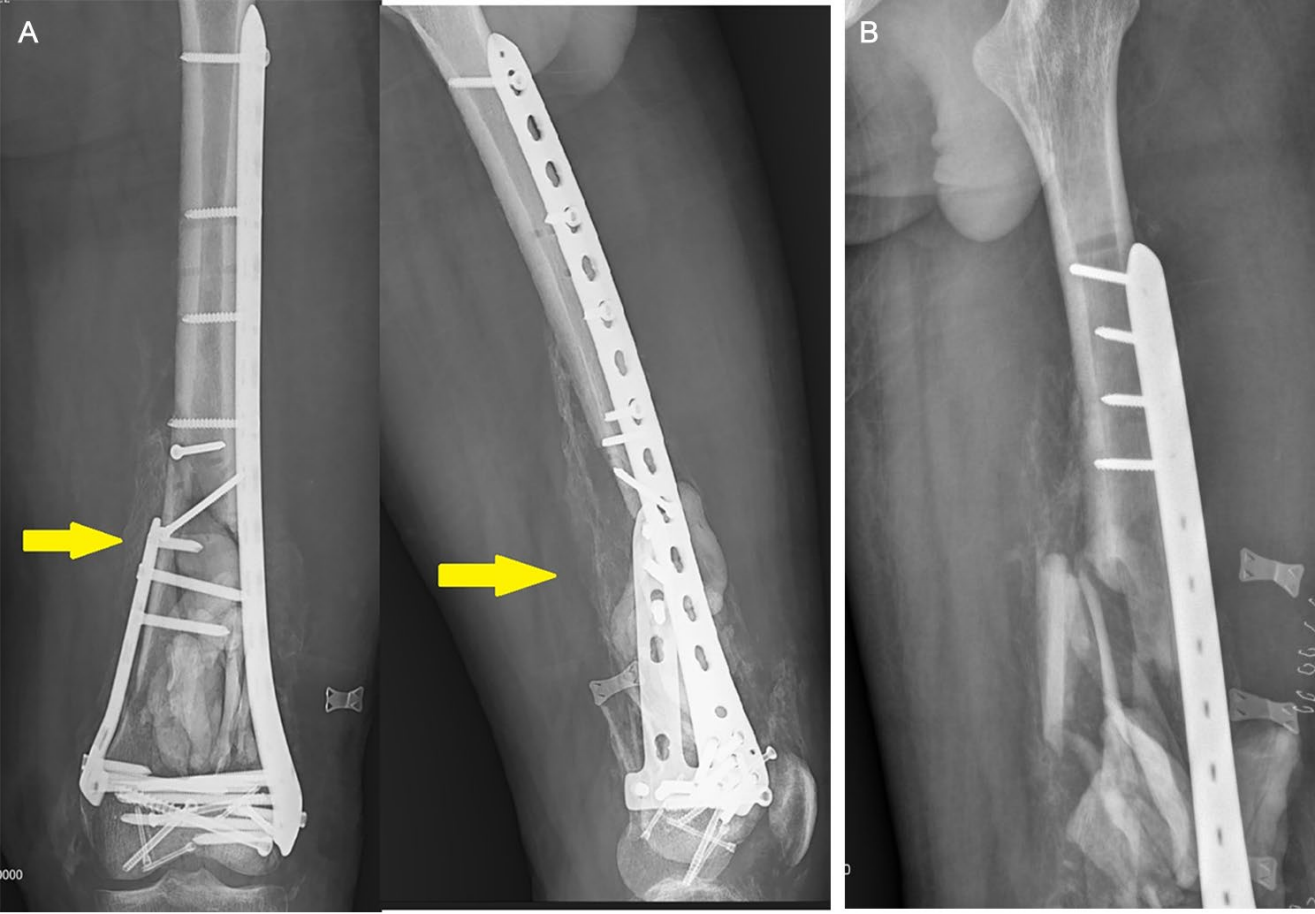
Hard callus formation in X-ray. (A) Patient One, (B) Patient Two
